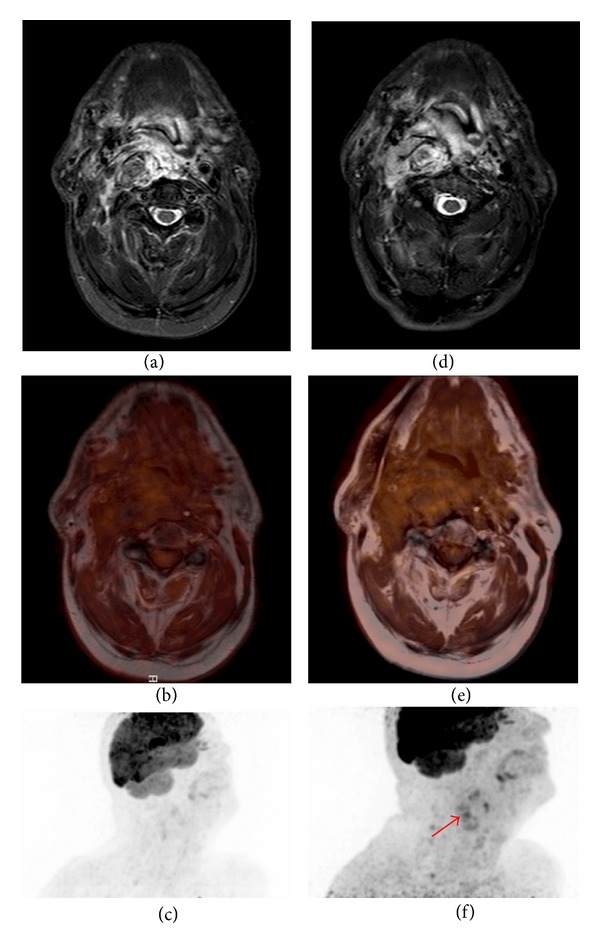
A 68-year-old patient with liposarcoma of the oropharynx. A maximum intensity projection (MIP) of the PET shows increased FDG uptake in the tumour ((f), arrow) as compared to the PET six months before (c). The short tau inversion recovery (STIR) images ((a) and (d)) and the corresponding fused PET/MR images ((d) and (e)) show no change in tumour size over six months.
